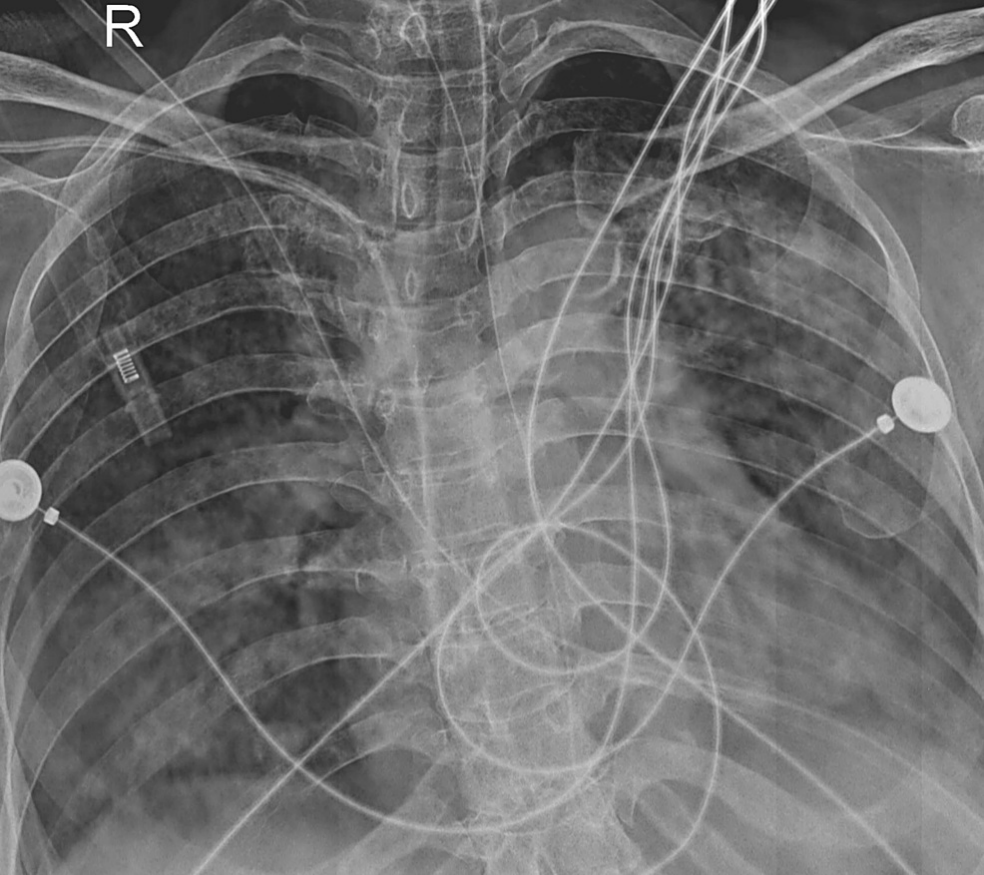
Chest X-ray on Day 6 of admission showed resolution.
